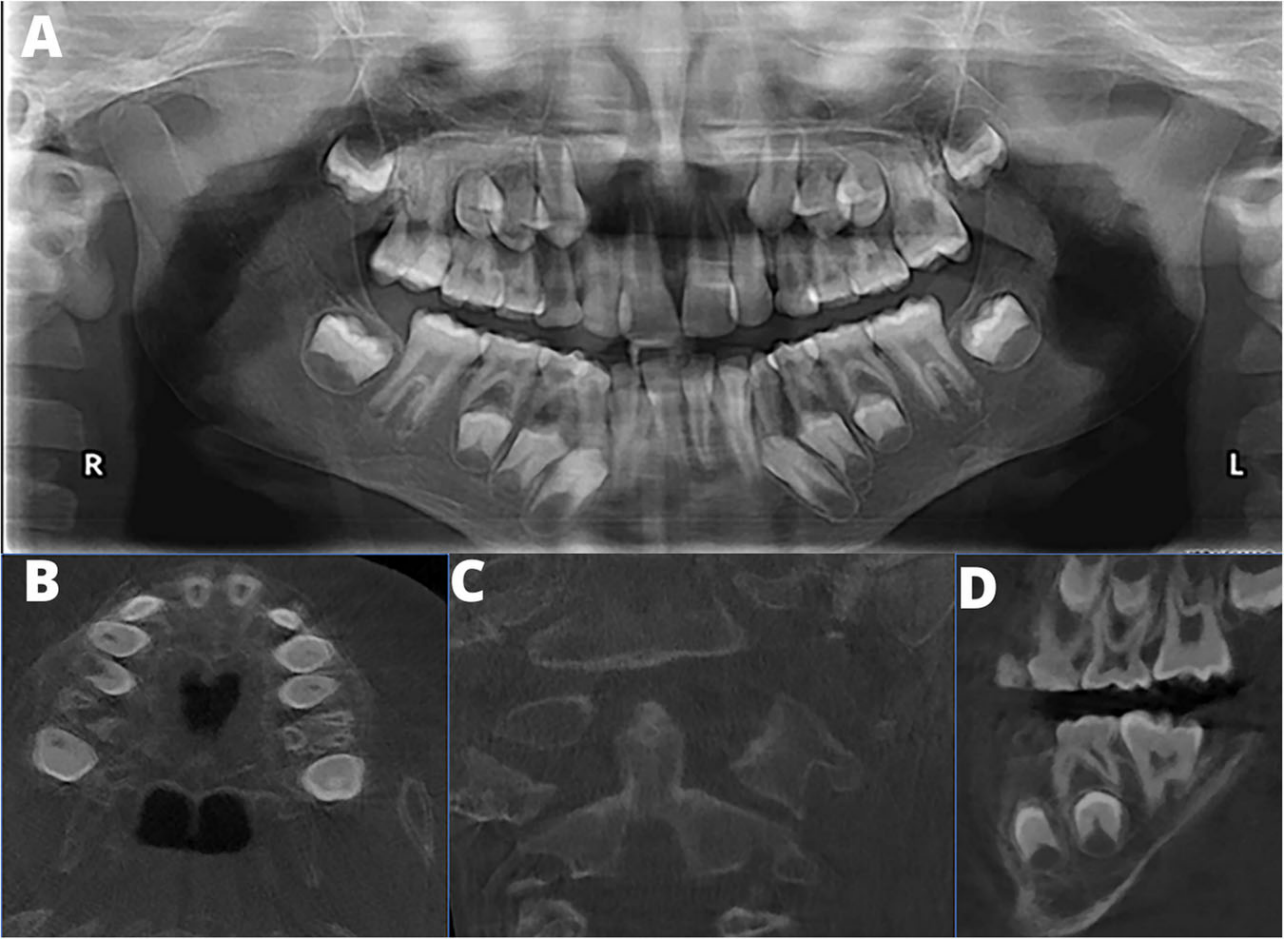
Craniofacial and oral radiographs of a 10-year old male with PD. A Panoramic radiograph of the affected boy. There is dental crowding and delayed exfoliation of several primary teeth. Mesotaurodontism of the upper and lower first permanent molars is evident. The mandibular condyles lack a constriction at the neck. B High-arched palate. C Abnormal shape and decreased density of C2. D Mesotaurodontism of his first permanent molars.
